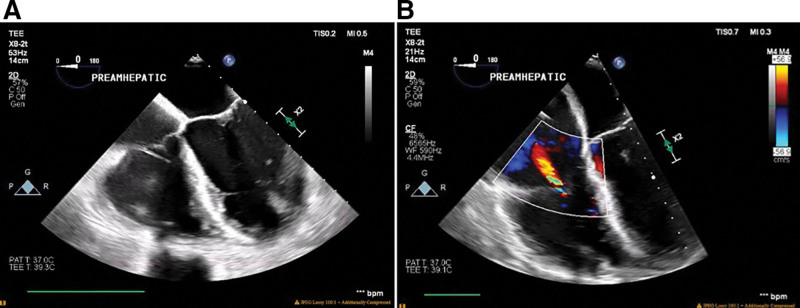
A and B, Intraoperative transesophageal echocardiography. RV dilation with preserved systolic function and mild TR on inhaled epoprostenol. Postreperfusion, RV function was hyperdynamic on epinephrine and vasopressin (not shown). RV, right ventricle; TR, tricuspid regurgitation.
